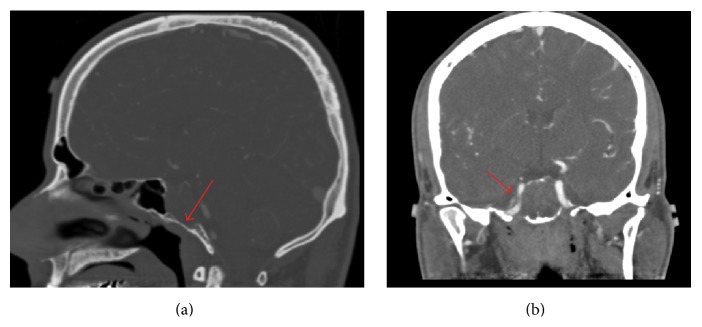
Preoperative computed tomography angiogram (CTA) scan, sagittal bone window (a) and coronal vascular window (b) sections, showing poorly vascularized clival mass that erodes the clivus (a) and encases the right internal carotid artery (b).
